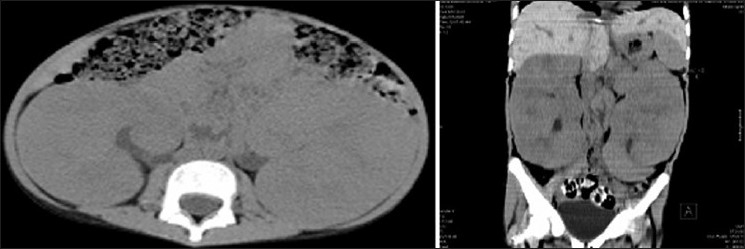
CT scan showing bilateral diffuse renal enlargement
